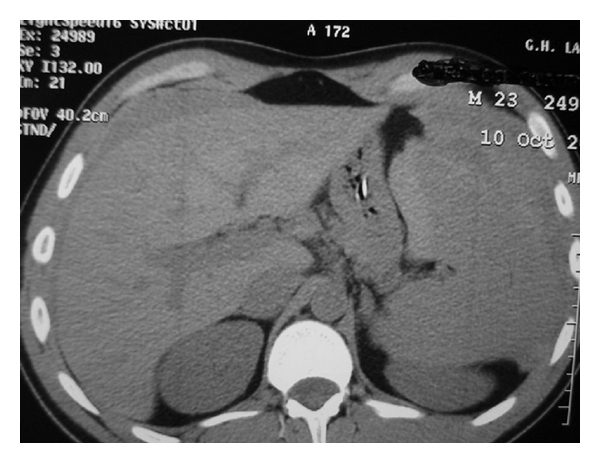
Preoperative CT scan indicative of the splenic rupture and hemoperitoneum.
